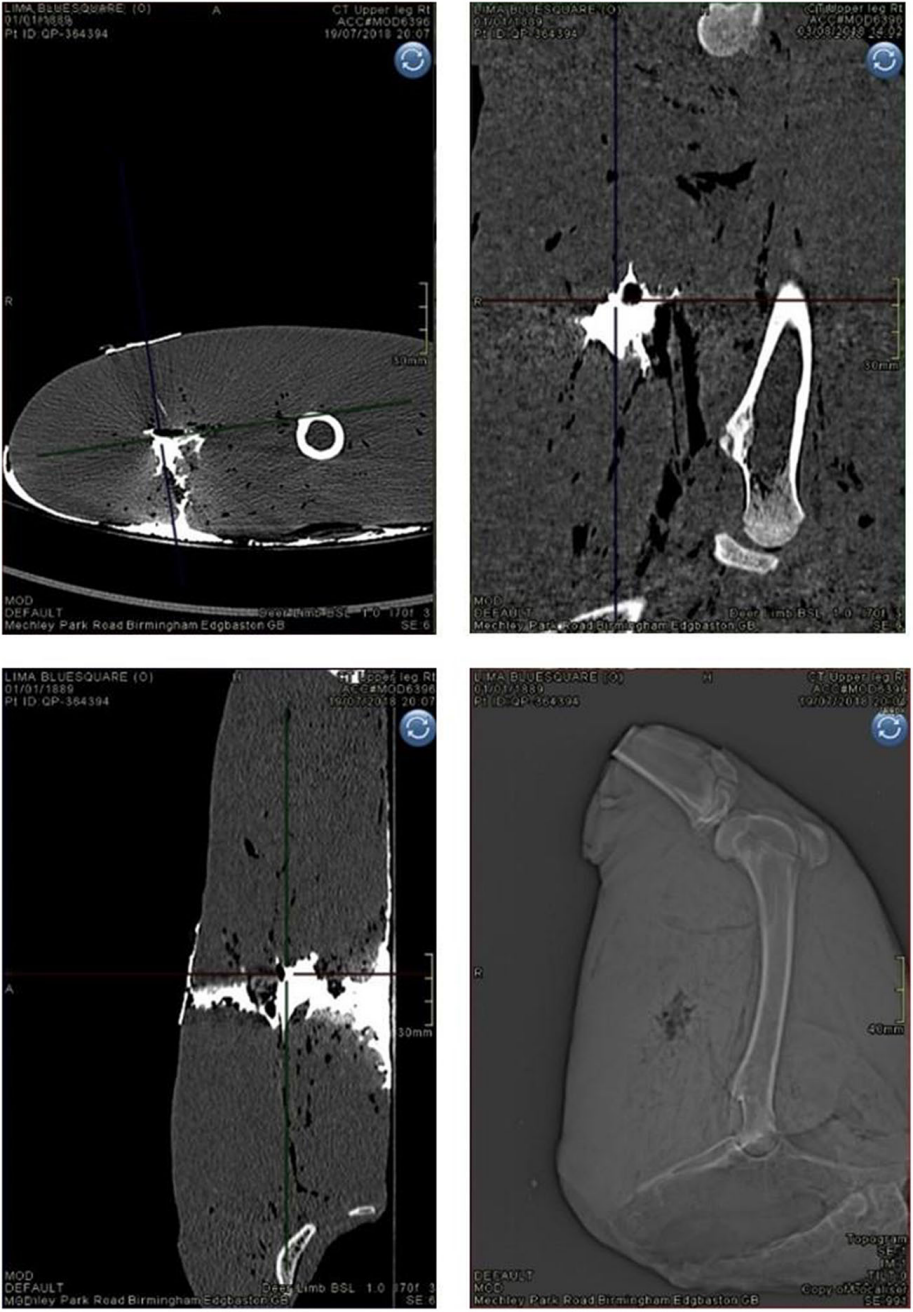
Clockwise from top left: contrast image, axial plane; contrast image, sagittal plane; CT scout view, sagittal plane (prior to contrast); contrast image, coronal plane
